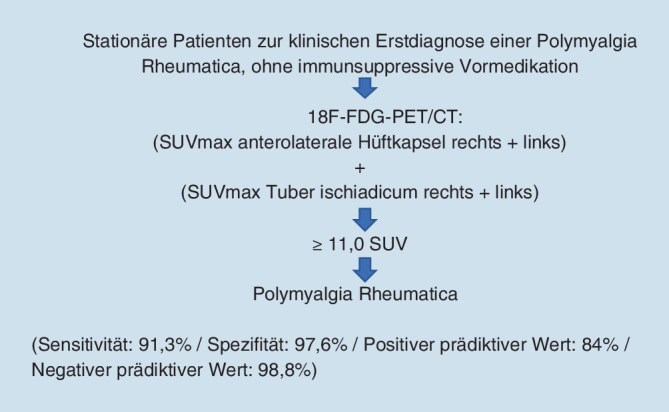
Unsere Analyse zeigte weiterhin, dass mit steigender Dauer der Immunsuppression vor der 18F-FDG-PET/CT die Spezifität dieser diagnostischen Methode zwar kontinuierlich sinkt, sie allerdings zum Zeitpunkt der Erstdiagnose dieser Erkrankung, vermutlich also im Frühstadium, immer bei über 90 % liegt.
